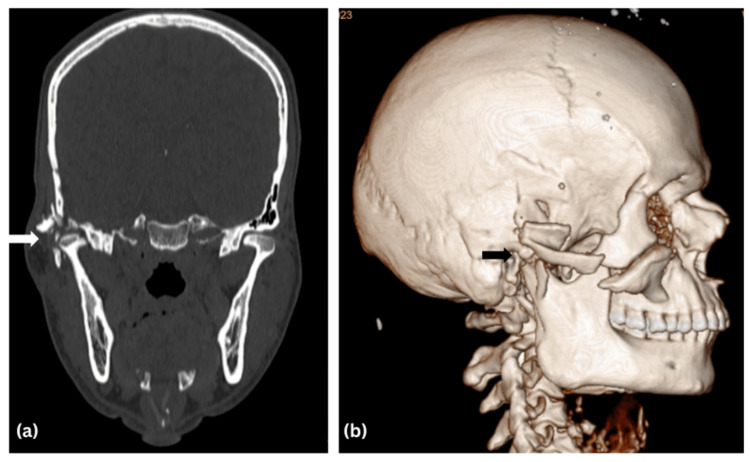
(a) Coronal view and (b) three-dimensional reconstruction of head CT showing the ankylotic mass over the right temporomandibular joint.
